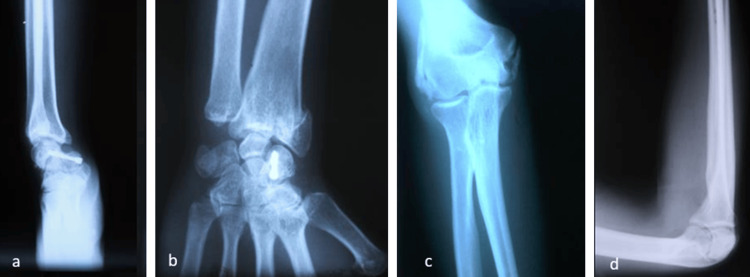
Radiographic images after one year of follow-up. (a-b) AP and profil imaging of the wrist and (c-d) AP and profil radiographs of the elbow.
